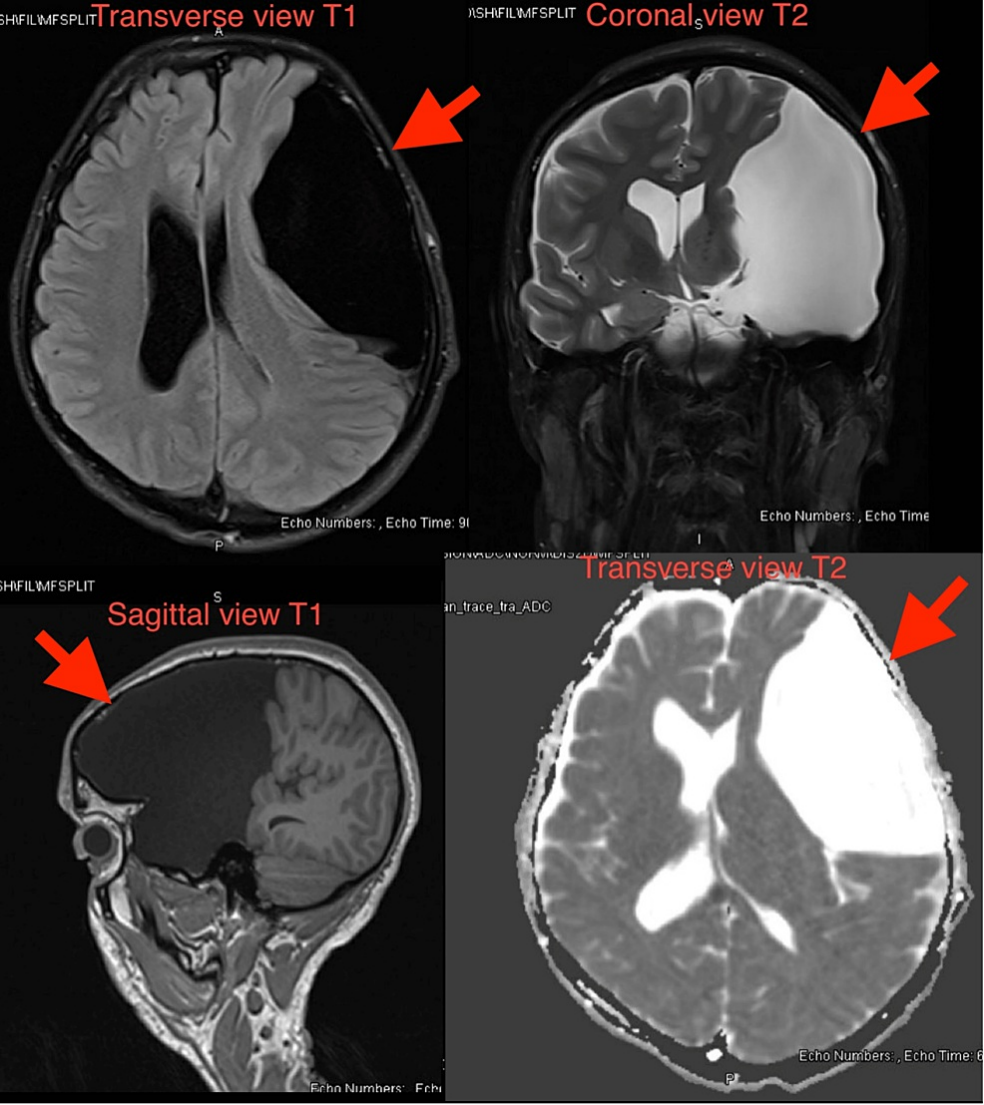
Multiple cuts from MRI head showing 6.5 x 11.5 x 12.5 cm left hemispheric arachnoid cyst with an 8.5 mm midline shift to the right MRI: magnetic resonance imaging
